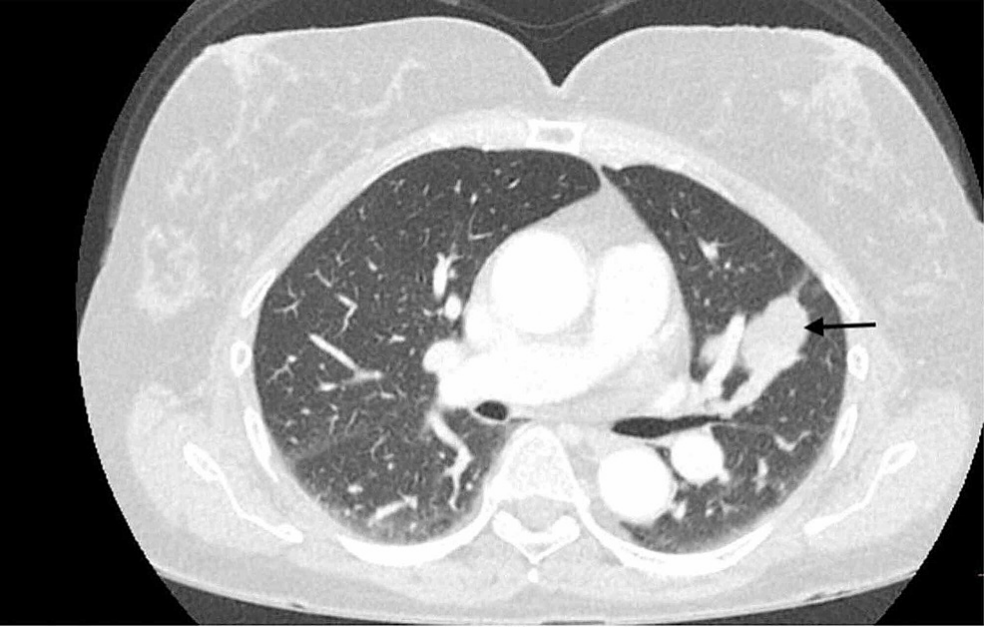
Computed tomography of chest showing a decrease in size of pulmonary nodules (black arrow) three months after starting nab-sirolimus.
